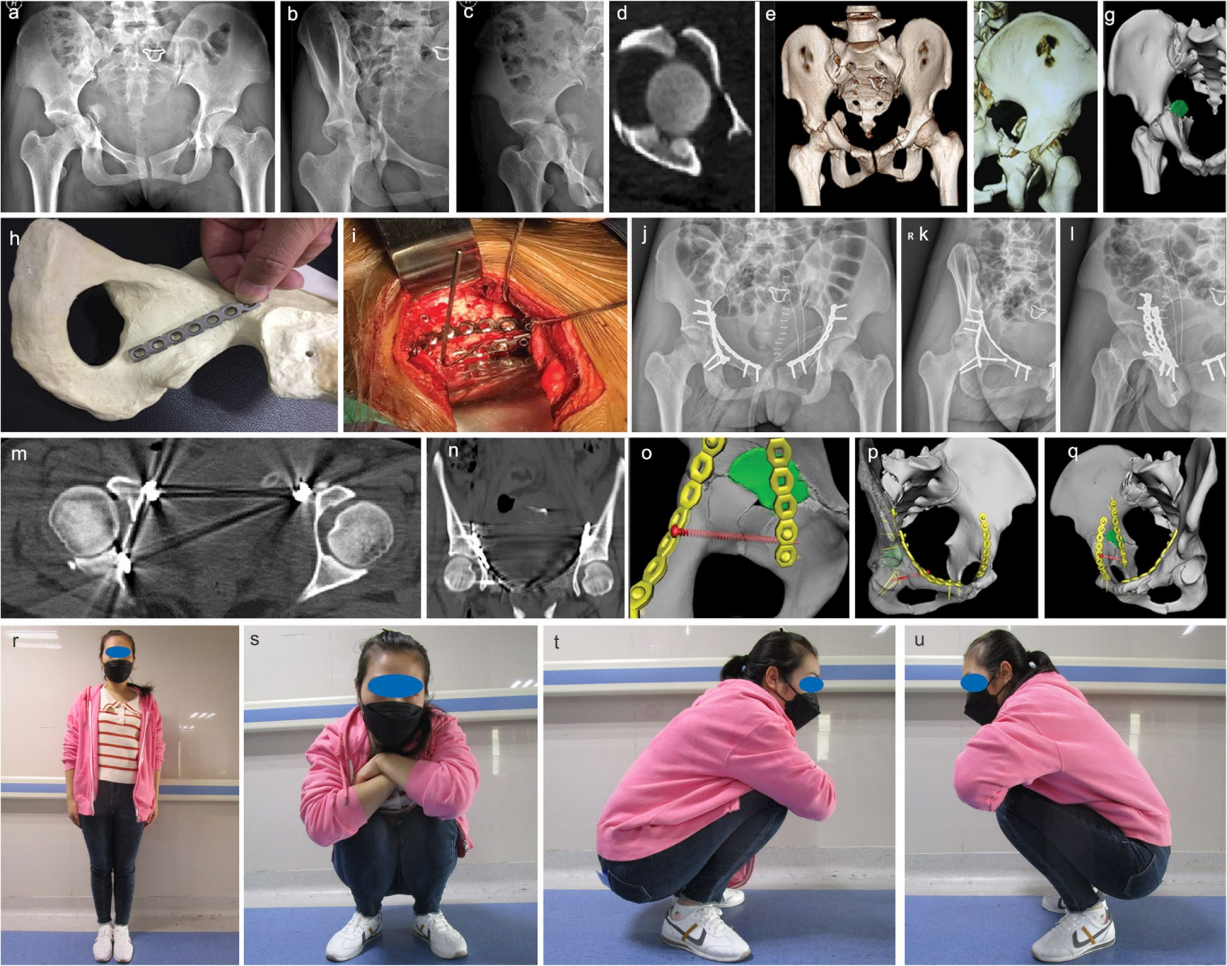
a–c X-rays of Case no. 1, who was involved in a traffic accident resulting in a right T-shaped acetabular fracture; d–g the free fragment of “square zone” and low level posterior column fracture line were demonstrated in three-dimensional reconstruction radiograph; h preoperative design of the placement of oblique-ilioischial plate; i reduction and fixation were performed with the oblique-ilioischial plate through a single modified Stoppa approach. Postoperative radiographs of the pelvis (anteroposterior) j, obturator oblique k, and iliac crest oblique l showed a satisfactory reduction of the fracture, and computed tomography images showed a good anatomic reduction of the fracture m, n. The three-dimensional reconstruction shows the location of the implant o–q. Photographs at 9-months postoperatively show that satisfactory hip function was achieved
